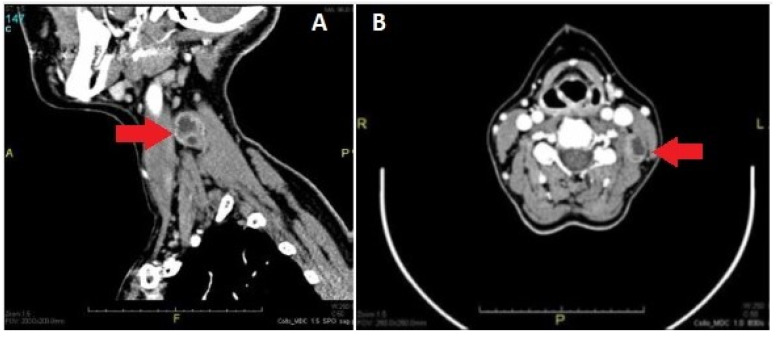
CT imaging of a vagal schwannoma (red arrow) on the left cervical side: (A) sagittal view; (B) axial view.
